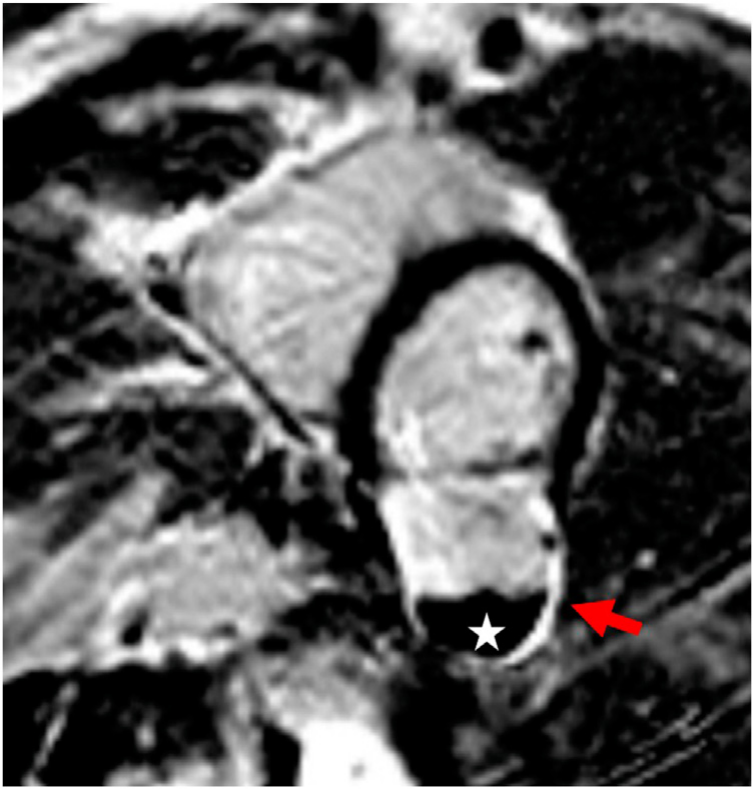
Cardiovascular magnetic resonance imaging, phase-sensitive inversion imaging, late gadolinium enhancing sequence, mid-LV short-axis display, demonstrates a markedly thinned, transmural late gadolinium enhancing inferolateral myocardial wall segment of the LVP (*arrow*) with a large, dependent nonenhancing filling defect (*star*) within its lumen consistent with thrombus.
